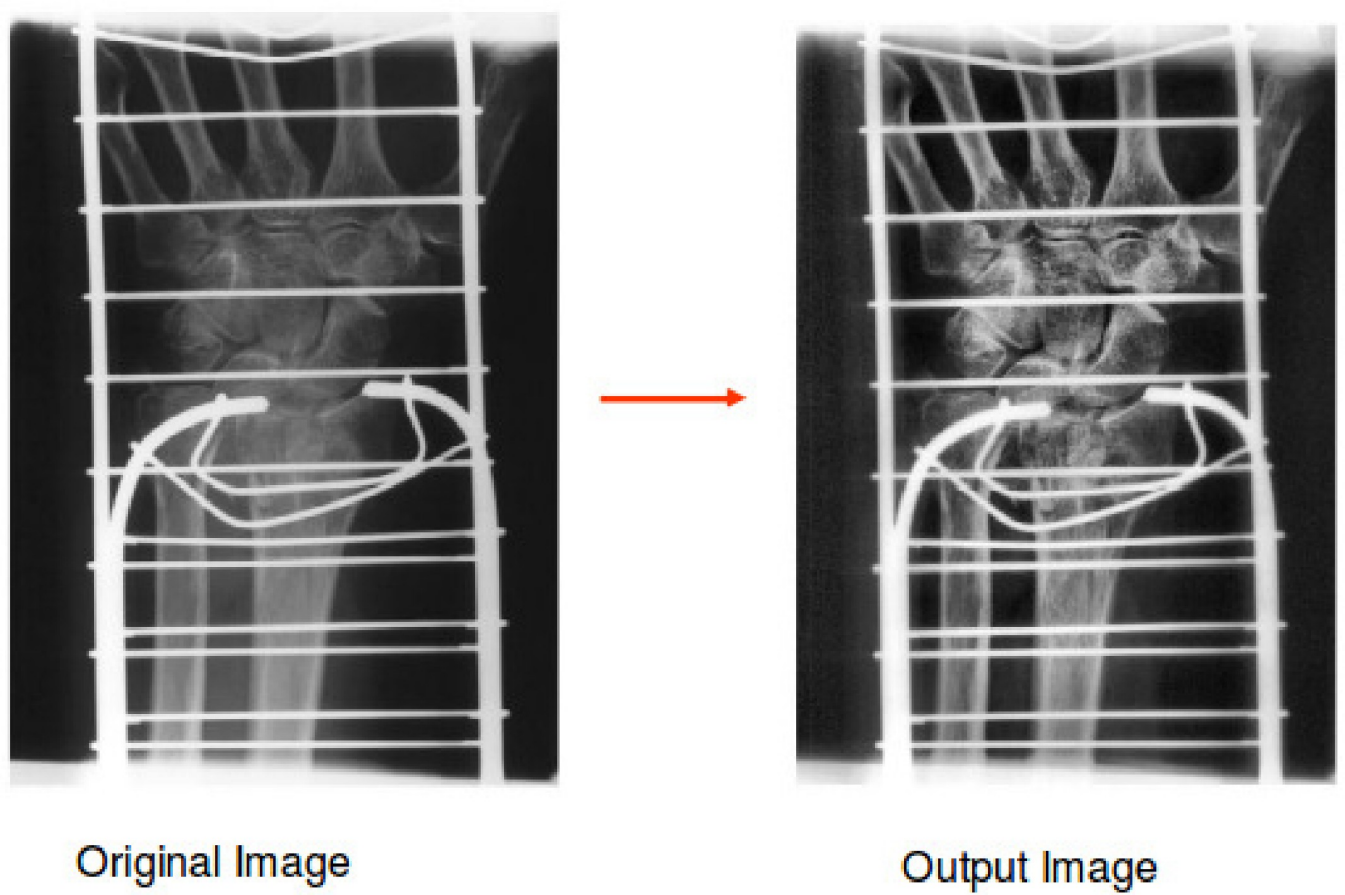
Medical image before and after Contrast Limited Adaptive Histogram Equation (CLAHE) was performed. The radiograph was randomly chosen from the ImageCLEF 2009 Medical Annotation Task Training Set. Republished from [21] under a CC BY license, with permission from[RWTH Aachen], original copyright [2009].
